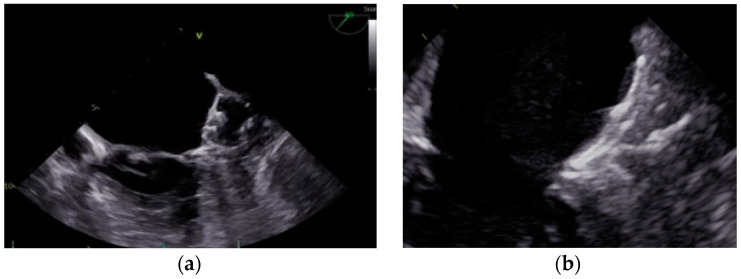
Percutaneous LAAO device categories. (a) Plug-based devices. Transesophageal echocardiogram. Midesophageal plane at 49°. Plug-based WATCHMAN FLX™ device. (b) Disc-/lobe-based devices. Transesophageal echocardiogram. Midesophageal plane at 73°. Disc-/lobe-based Amplatzer™ Amulet™ device.
